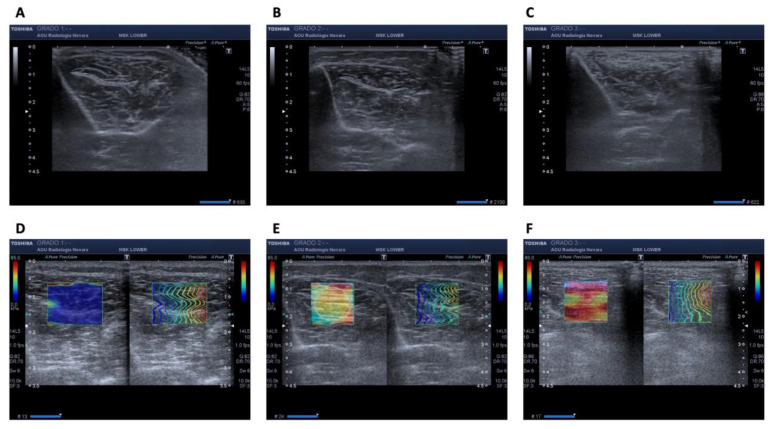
Tibialis anterior muscle ultrasound assessment in terms of echogenicity, grade 1 (A), grade 2 (B), and grade 3 (C), and stiffness, measured with shear wave elastography with grade 1 (D), grade 2 (E), and grade 3 (F).
